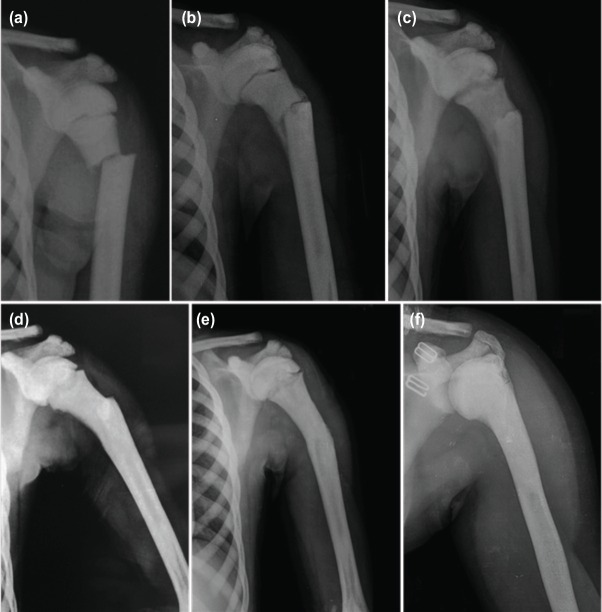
Serial radiographs of the patient’s through-out the follow-up controls at (a) initial admission, (b) 1 month, (c) 2 months, (d) 6 months, (e) 48 months and (f) at the final follow-up seven years after the initial injury. The remodeling of fracture is clearly seen.
